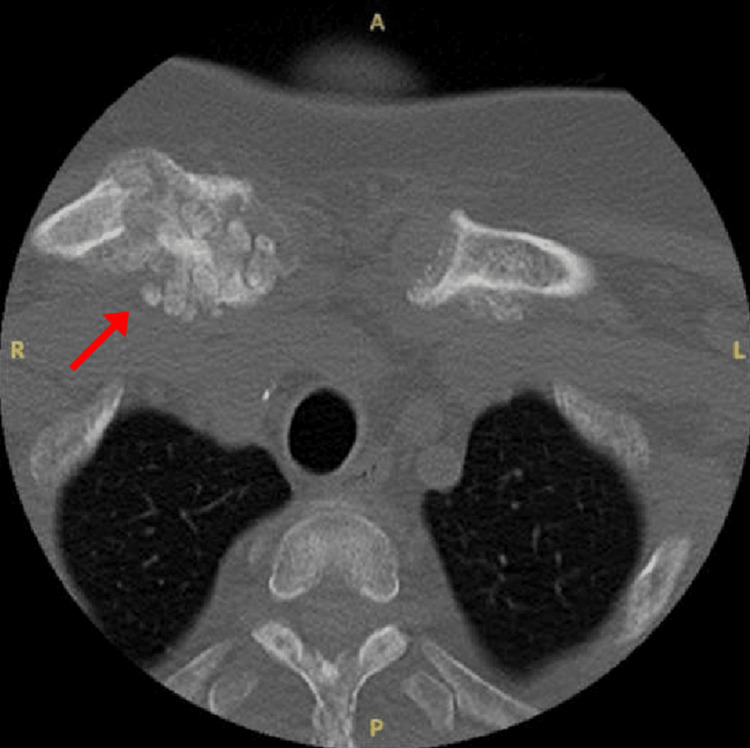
CT axial view of the sternoclavicular region.
